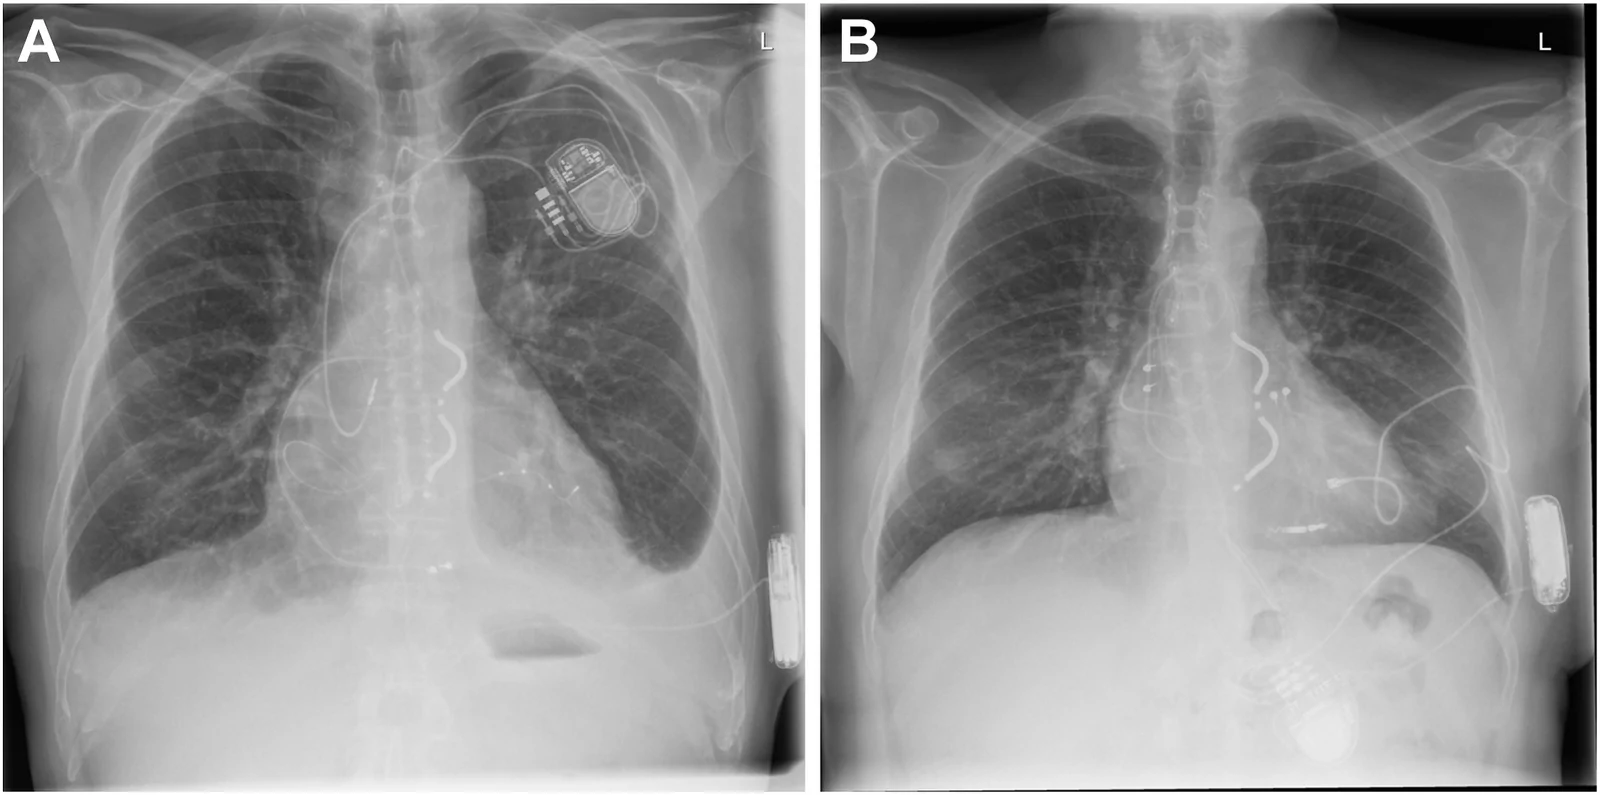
A and B: Radiograph after implant for both patients.
